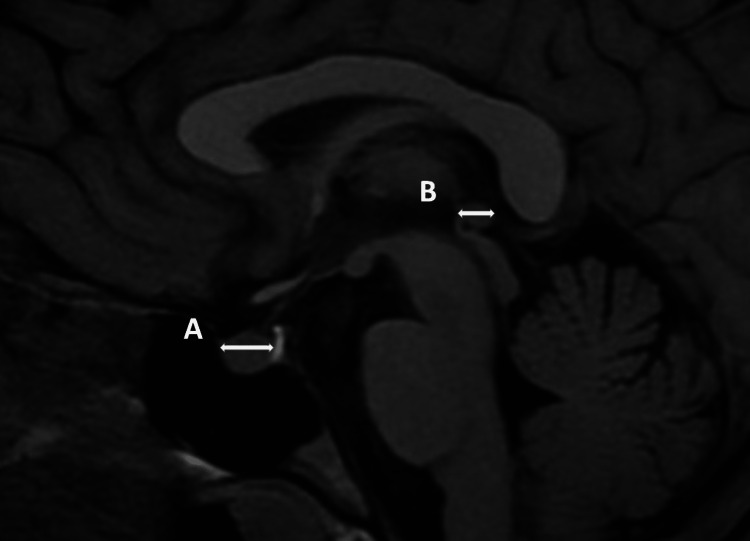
Sagittal MRI images demonstrate the height and anteroposterior length measurement of the pituitary gland (A) and the pineal gland (B).
